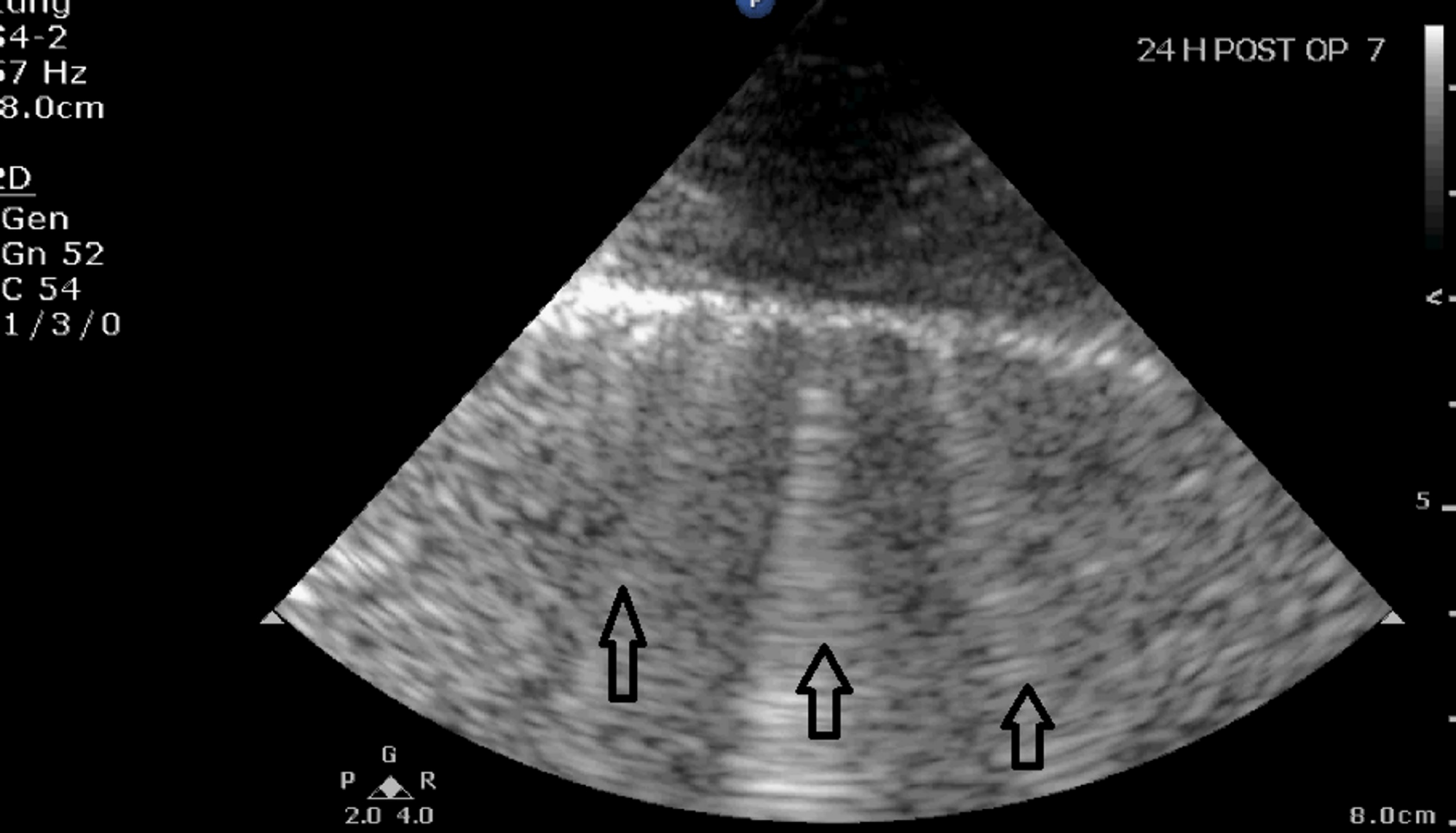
Lung ultrasound image showing B-lines (arrows) 24 hours after cardiac surgery
